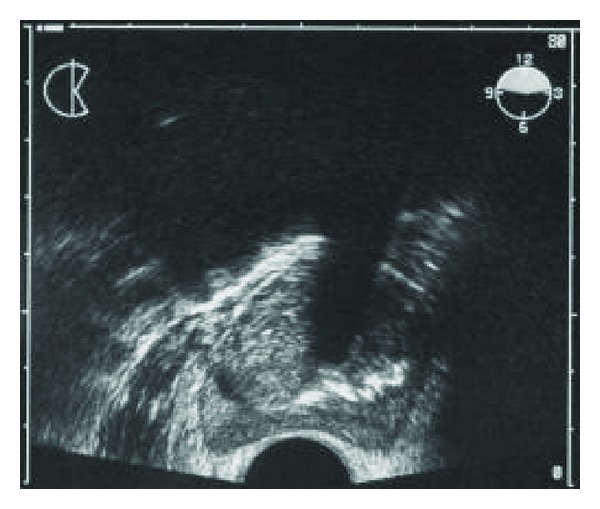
Transrectal ultrasound: hypoechoic lesion posterior and cranial to the prostate.
